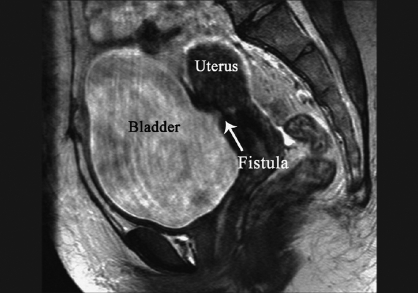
Sagittal T2-weighted HR/SENSE MR image (TR/TE, 4774/90) shows abnormal hypointense area (*arrow*) in the posterior wall of bladder communicating with uterus above the isthmus
